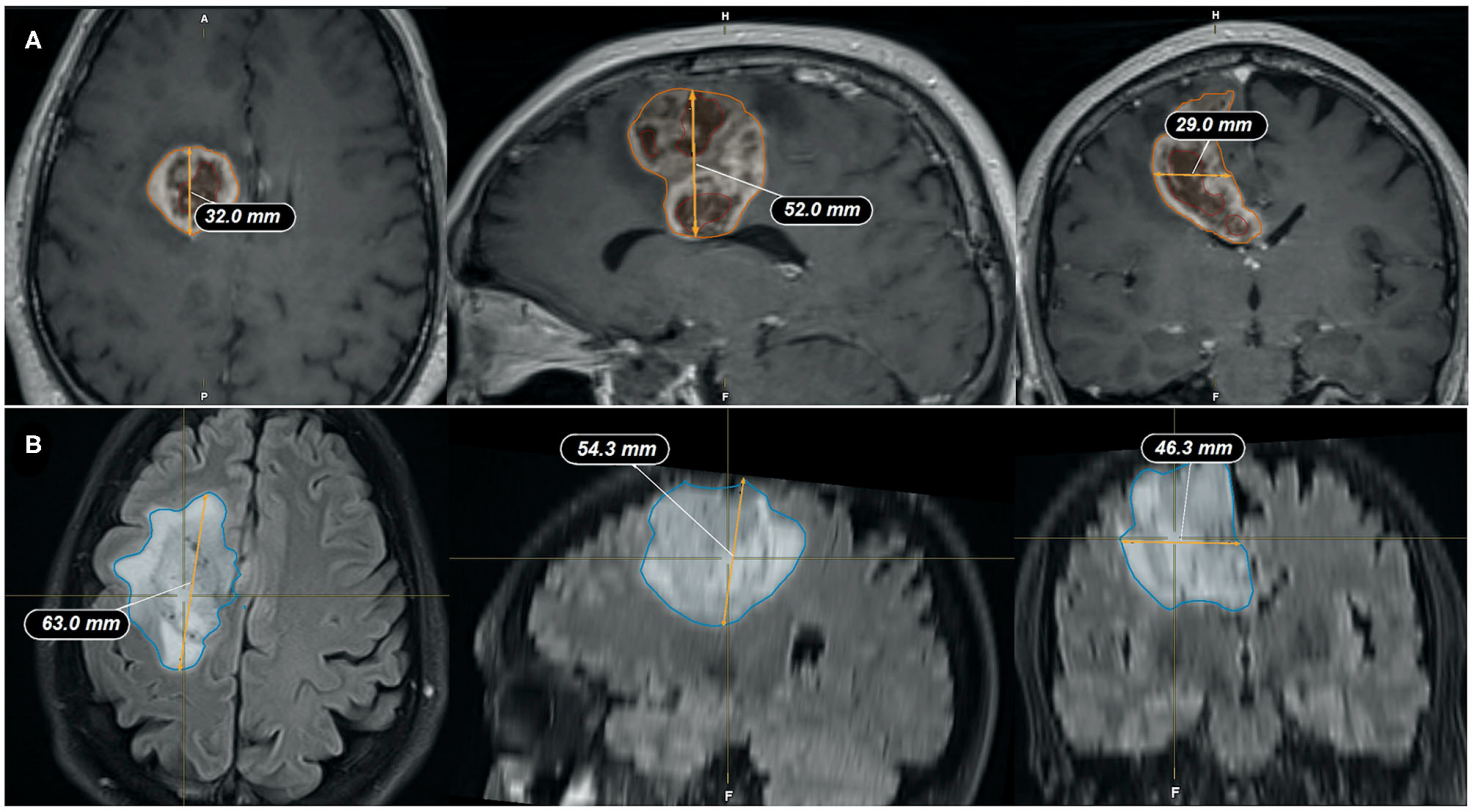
Representative measurements of the tumor size by the ellipsoid method. (A) Tumor mass volume is: (32 × 52 × 29 mm)/2 = 24.13 cm^3^; (B) FLAIR-T_2_ volume is: (63 × 54.3 × 46.3 mm)/2 = 79.19 cm^3^. Coronal (left), Sagital (center), and Axial (right) slices through the tumor.
